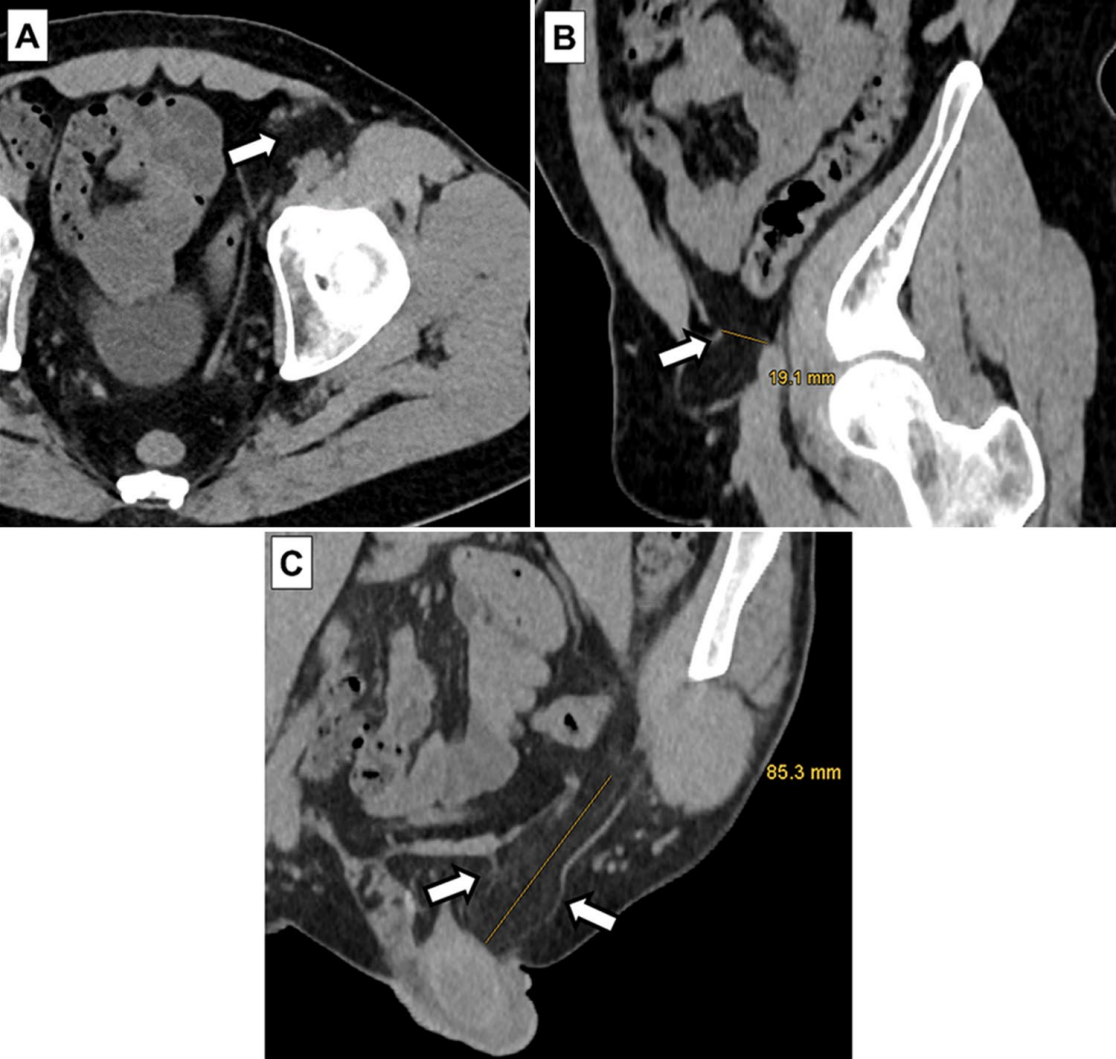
Axial (A), sagittal oblique (B) and coronal oblique (C) CT images of a 49-year-old male patient with a fat-containing indirect inguinal hernia on the left side. Hernia neck size (arrows in A and B) measured 19.1 mm and the maximum diameter of the hernia sac (arrows in C) was 85.3 mm
